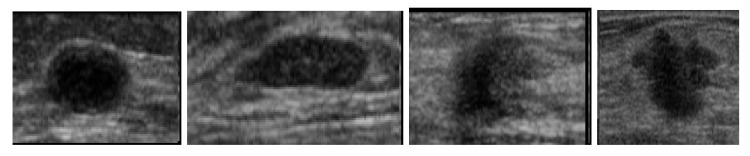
Four examples of breast ultrasound images with lesions in a darker gray scale nearby the center of the images.
